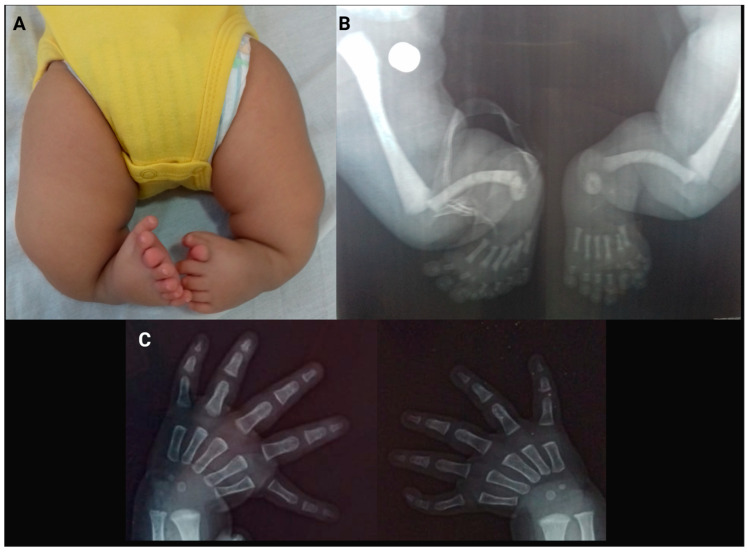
Lower limbs. Photograph (A) and X-ray bone radiographic imaging (B) showing bilateral tibial agenesis of our patient. Hand X-rays (C) showing bilateral triphalangeal thumb. Created with BioRender.com.
